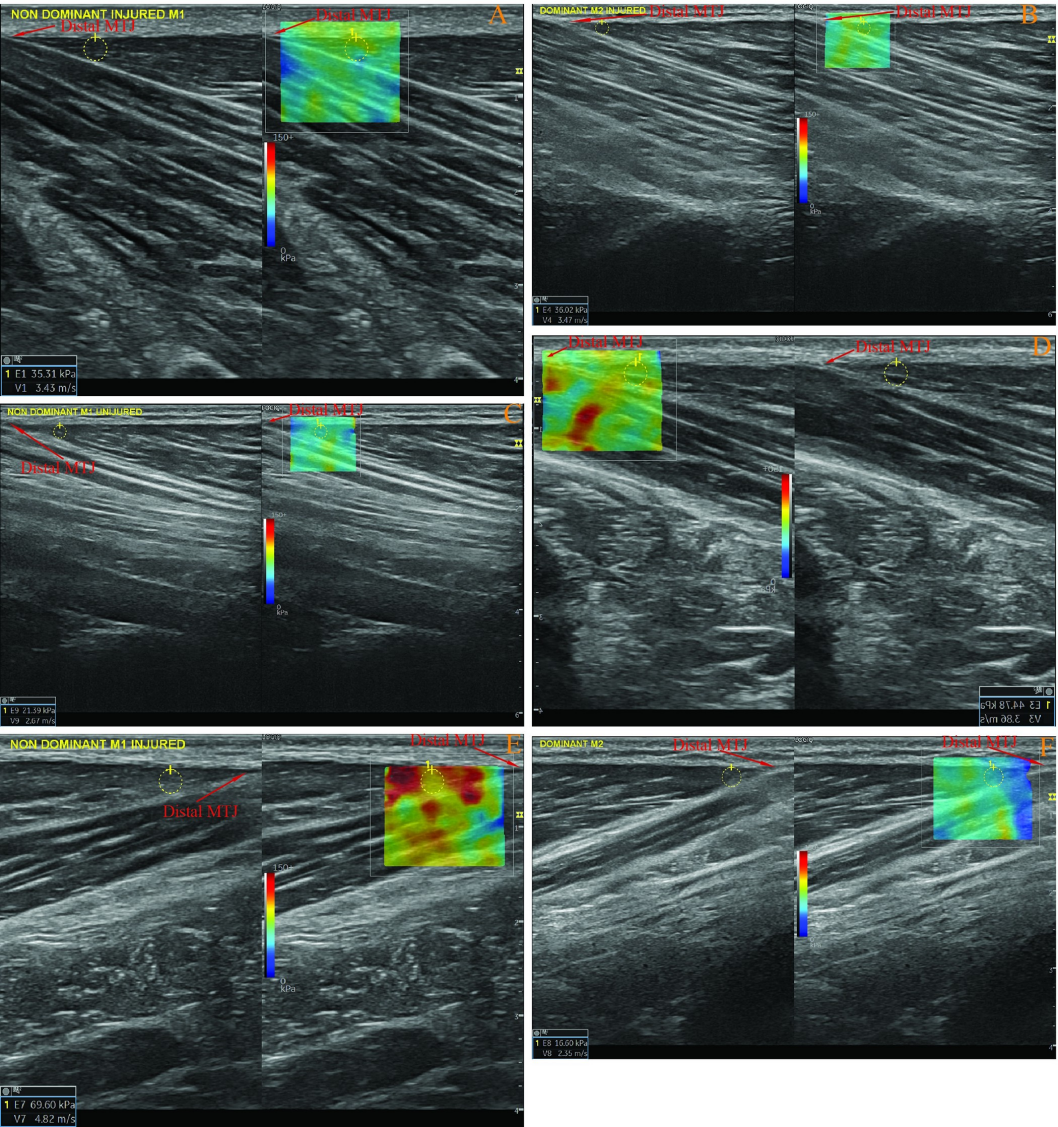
A-F: Random examples of the measurements of distal musculotendinous junction passive stiffness of the biceps femoris long head via ultrasound-based shear-wave elastography in various participants. The different image orientations, whether from left to right or vice versa, result from the 180° differences in the grip positions of the ultrasound probes during each measurement, which do not affect the outcomes.
